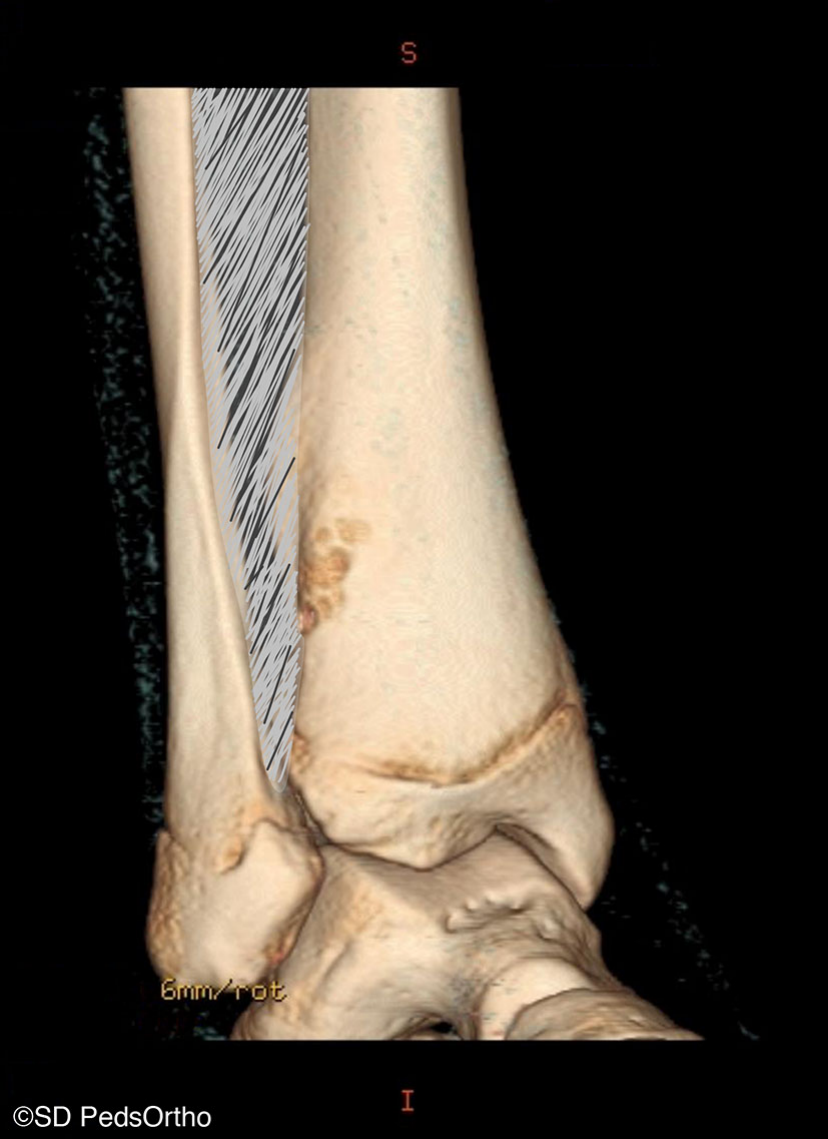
Three-dimensional reconstruction of computed tomography (CT) scan revealing an NOF with cortical breach located anterolaterally in the distal tibia
